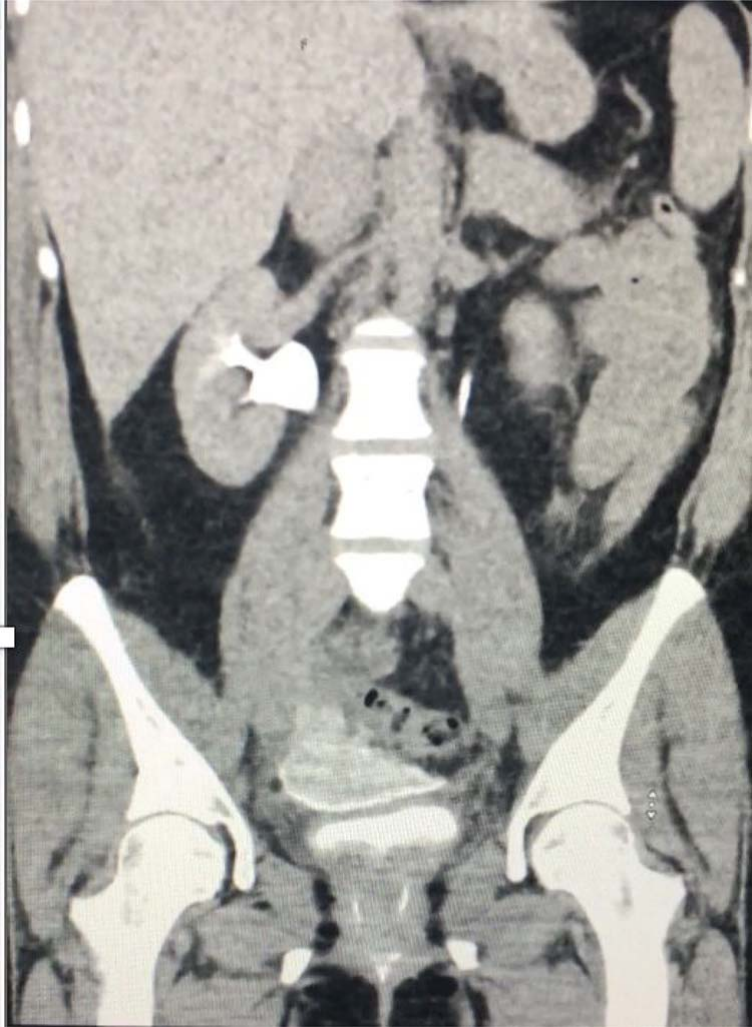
Coronal delayed phase CT urogram showing pooling of contrast in the pelvis.
